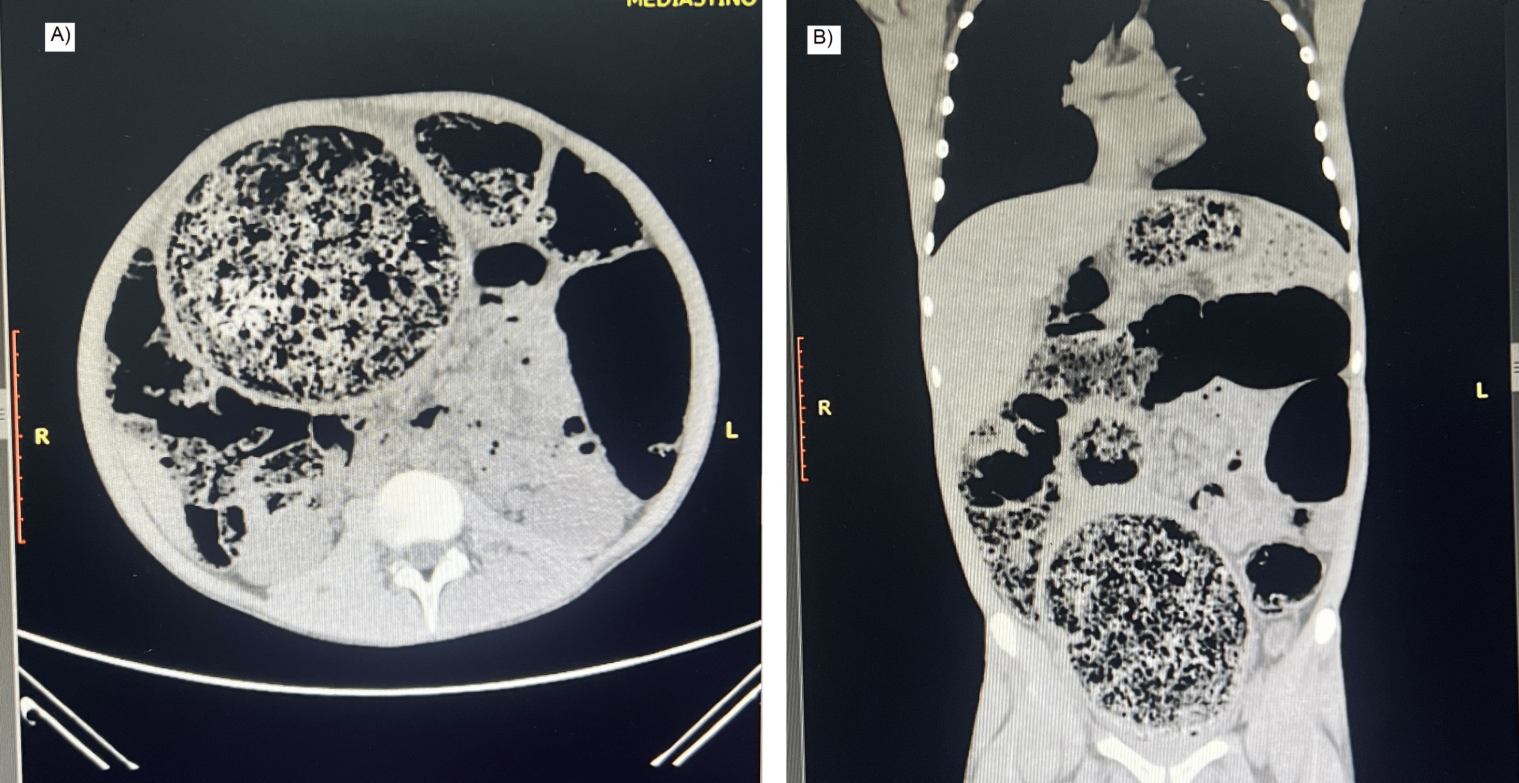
CT of the abdomen showing massive colonic dilation and fecal impaction (A) Axial view demonstrating marked distension of the colon with a large fecaloma. (B) Coronal reconstruction confirming diffuse colonic dilation and extensive fecal material throughout the colon.
